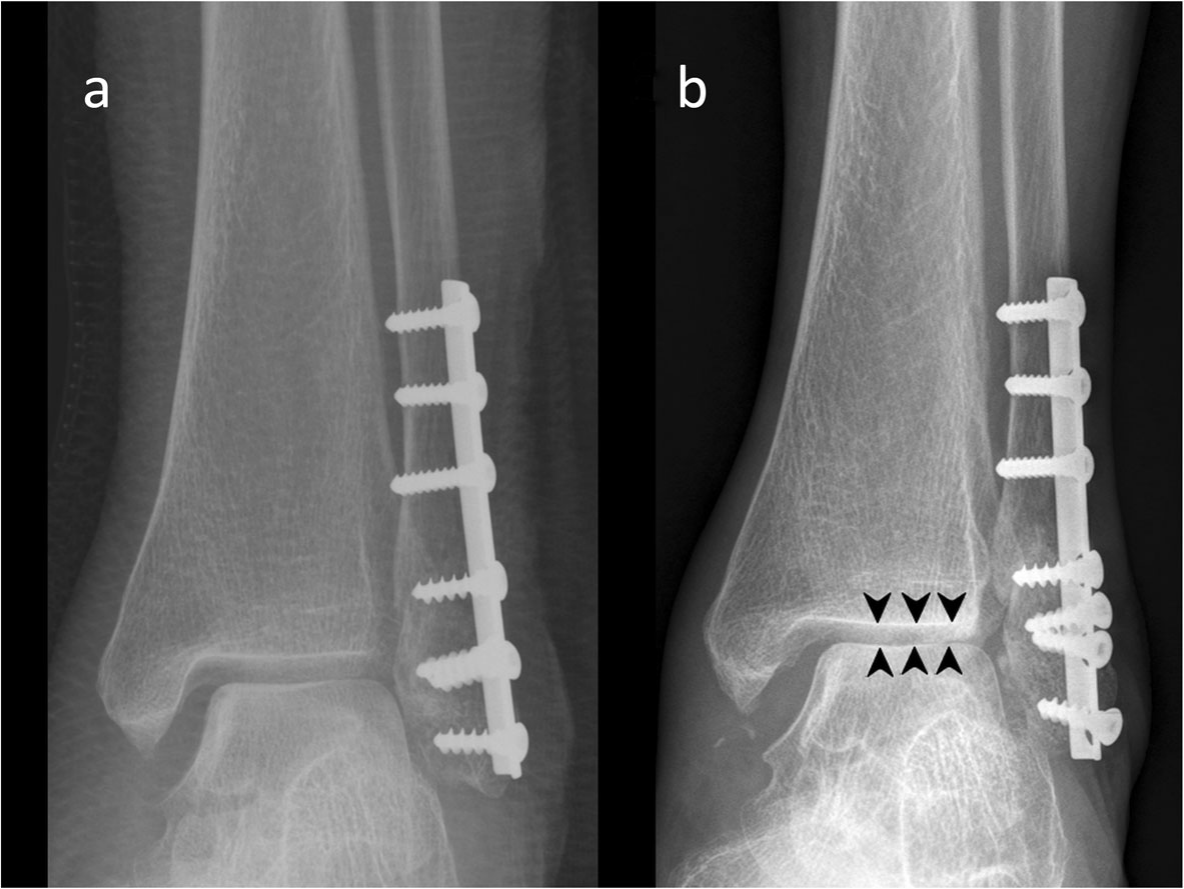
This 78-year-old woman was treated with an interfragmentary screw and a TP. During securing of the interfragmentary screw, the far cortex was cracked, and absolute stability was not achieved. The fibula length could not be anatomically maintained using a TP that was not a fixed angle device. a Finally, the reduction accuracy was fair (immediately postoperative radiograph). b One year later, it was malunited (the medial ankle-joint substantially widened), the OA grade worsened (arrowheads), and all distal screws were loosened
